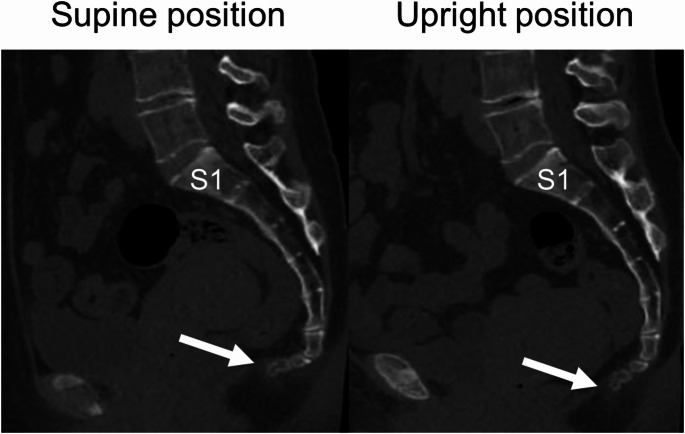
Sagittal CT images of the coccyx in a 60-year-old parous, healthy female volunteer in the supine (left) and upright (right) positions. In the supine position, the coccyx appears shorter and more curved, with anterior–superior displacement of the coccygeal tip (white arrow) compared with the upright position
